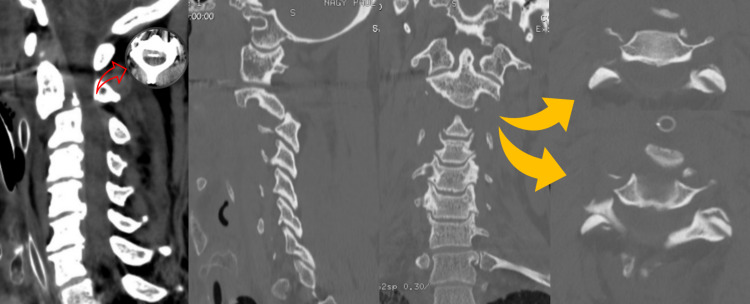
Cervical CT scan Left to right: Sagittal soft tissues (red arrow shows spinal cord compression between the C2-C3 segment), sagittal bone, coronal, and axial (yellow arrows show dislocated facets, reverse “hamburger bun” sign)
